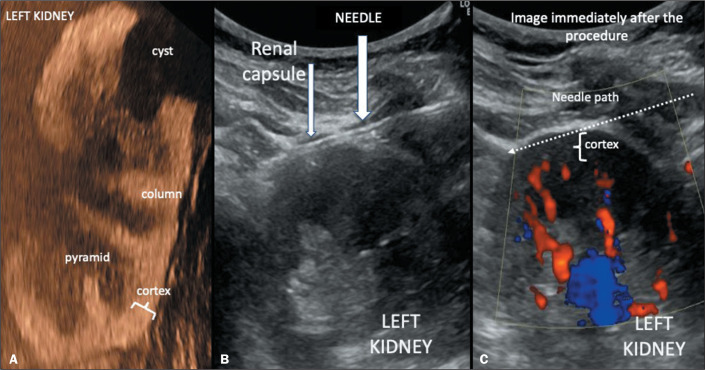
A: Arterial-phase ultrasound image with microbubble contrast showing
